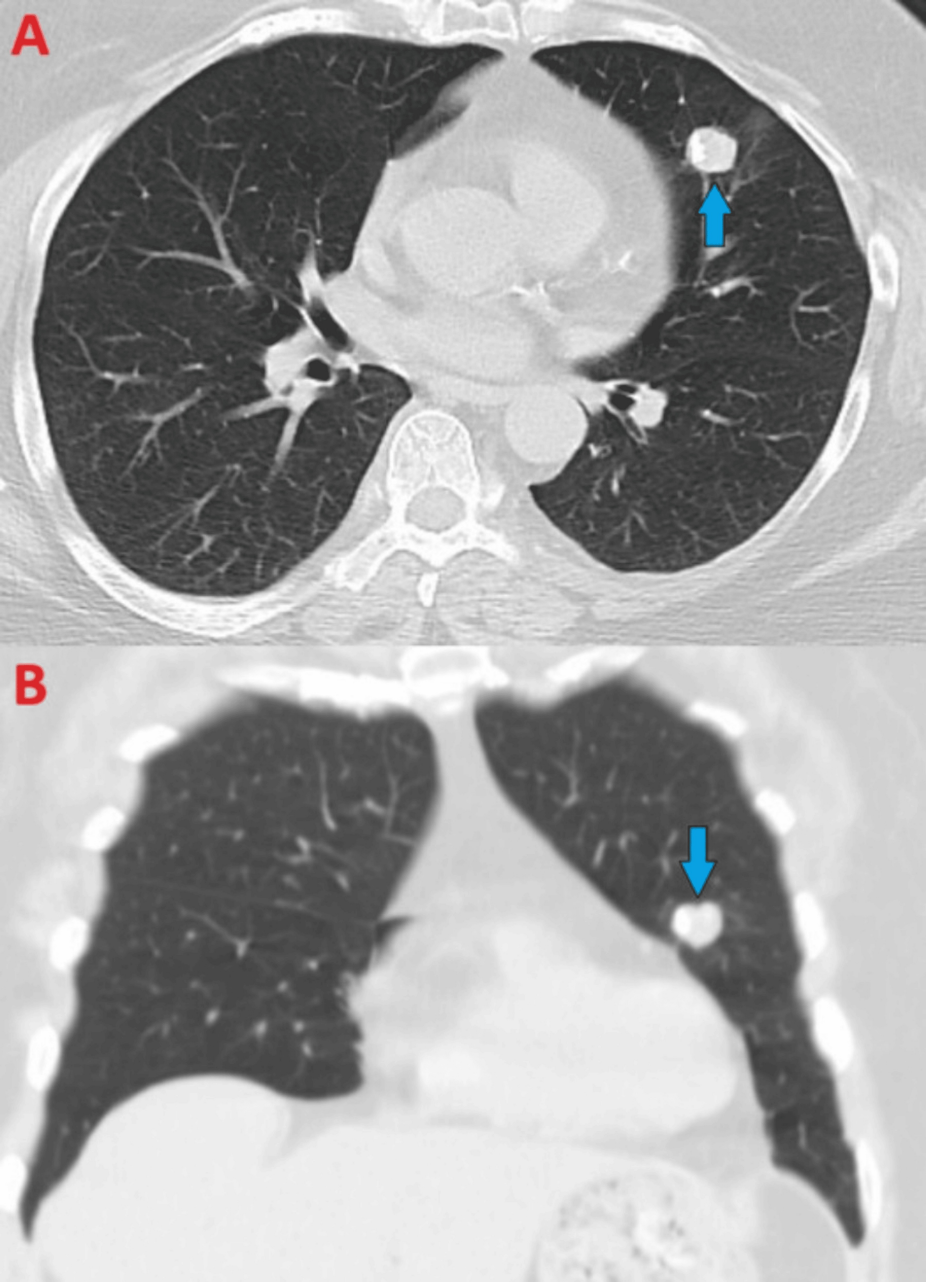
Chest CT (lung windows) demonstrating left circumferential lung nodule with eccentric calcification measuring 1.7 x 1.8 cm in axial view (A, blue arrow) and coronal view (B, blue arrow).
